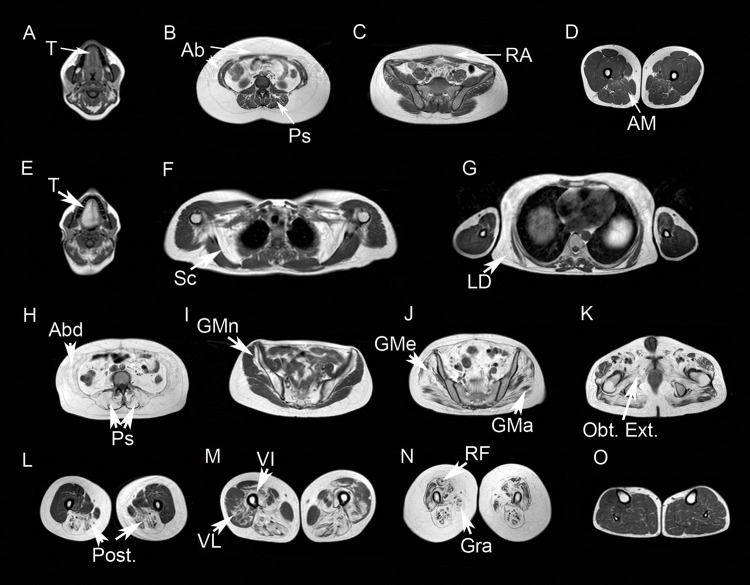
T1w Muscle MRI of patients with Pompe disease. We observed mild fatty infiltration in muscles of hyperCKemia patients: Tongue (Tin A), paraspinal muscles (Ps in B), lateral abdominal muscles (Ab in B) and *rectus abdominis* (RA in C) and in *adductor major* muscles (AM in D). The fatty infiltration in symptomatic patients was more evident (E-L). The muscles more commonly involved were tongue (T in E), *subscapularis* (Sc in F), *latissimus dorsi* (LD in G), the abdominal (Ab in H) and paraspinal muscles (Ps in H), the *Gluteus minor* (GMn in I), *medius* (GMe in J) and *maximus* (GMa in J), the muscles of the pelvic floor such as the *Externus Obturator* (Obt. Ext. in K), the posterior muscles of the thighs (Post in L), and the vasti muscles including *vastus intermedius* (VI in M) and *vastus lateralis* (VL in M). We observed involvement of *rectus femoris* and *gracillis* in advanced patients (RF and Gra in N). Muscles of the lower legs were commonly spared (O).
